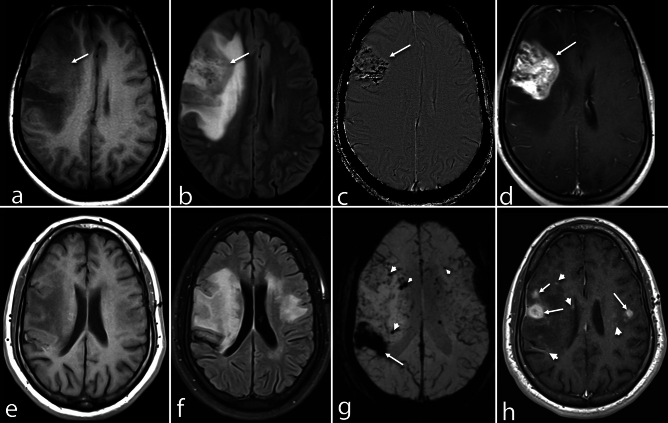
Atypical PCNSV patterns. (a–d) A 28-year-old woman presented with sudden onset left hemiparesis. Axial T1-WI (a) and FLAIR image (b) show a poorly defined heterogeneously mass lesion (arrows), isointense in T1-WI and hyperintense in FLAIR (b) in the right frontal lobe with adjacent edema causing mass effect on right lateral ventricles. Axial SWI phase image (c) shows multiple foci of microhemorrhages (arrow) in the lesion. In post-contrast study (d), the lesion shows intense heterogenous enhancement (arrow). (e–h) An 18-year-old boy with a history of progressive cognitive decline and headaches presented with sudden onset left hemiparesis. The axial T1-WI (e) and FLAIR image (f) show well-defined signal abnormalities, hypointense on T1-WI and hyperintense in FLAIR image, in the bilateral frontoparietal lobes' subcortical and periventricular white matter. The axial SWI image (g) shows macrohemorrhages (arrowheads) in the right parietal lobe with foci of microhemorrhages (arrows) in bilateral cerebral hemispheres. Axial post-contrast image (h) shows the nodular (arrowheads) and dot-linear (arrows) patterns of enhancement.
